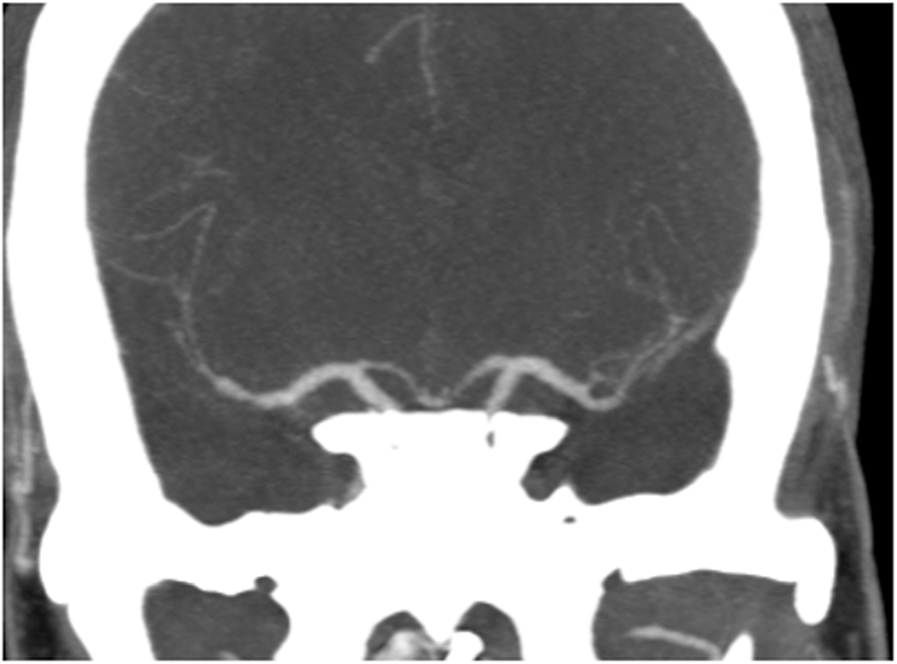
A 24 h CTA showed persistent vasodilatation of the vessel segments treated using the Cascade
